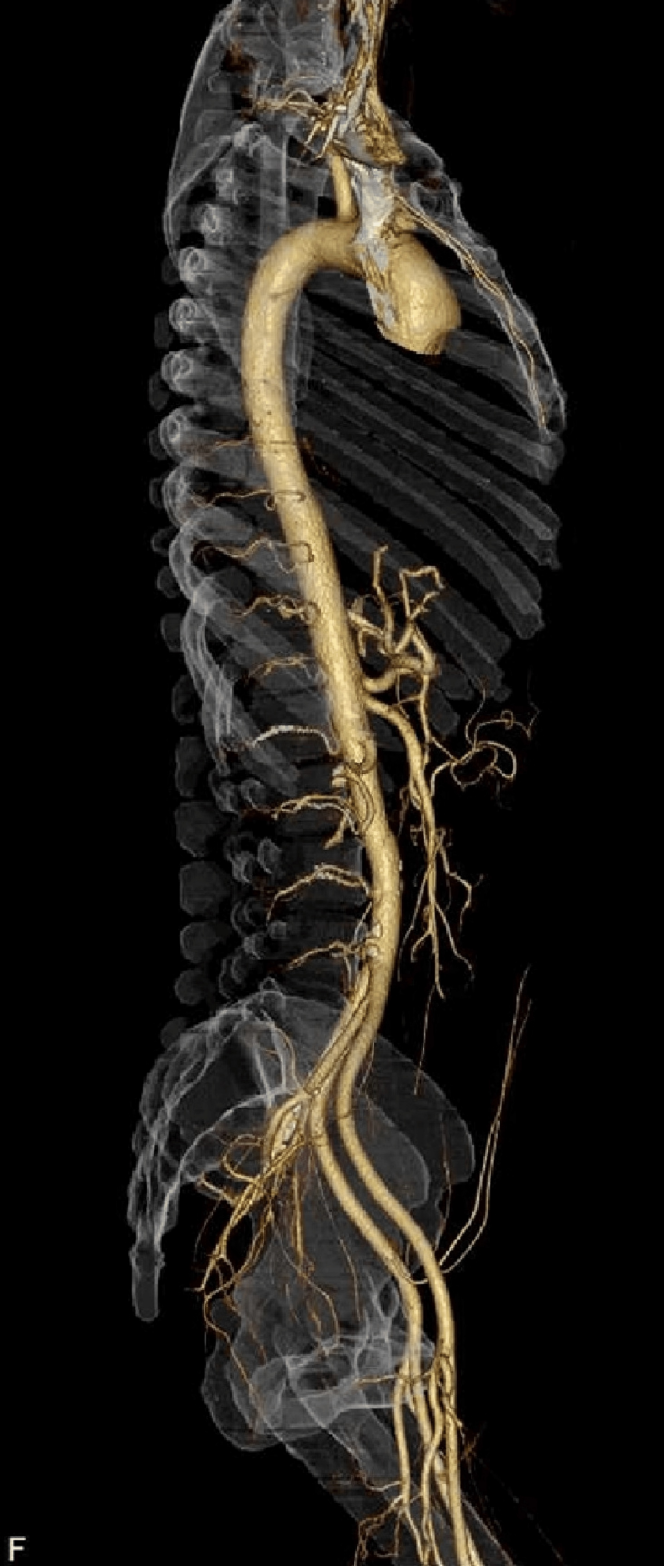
The result of three-dimensional computed tomography (CT) angiography performed on day six. CT angiography shows no abnormalities in the aorta.
